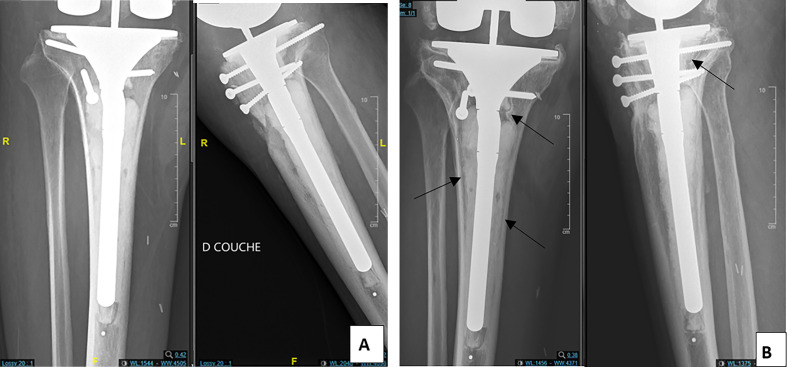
(A) Anteroposterior and lateral post operative radiographs of tibial long stem. (B) Anteroposterior and lateral radiographs of tibial long stem showing radiolucent lines (arrows) and loosening at 5 years follow-up.
